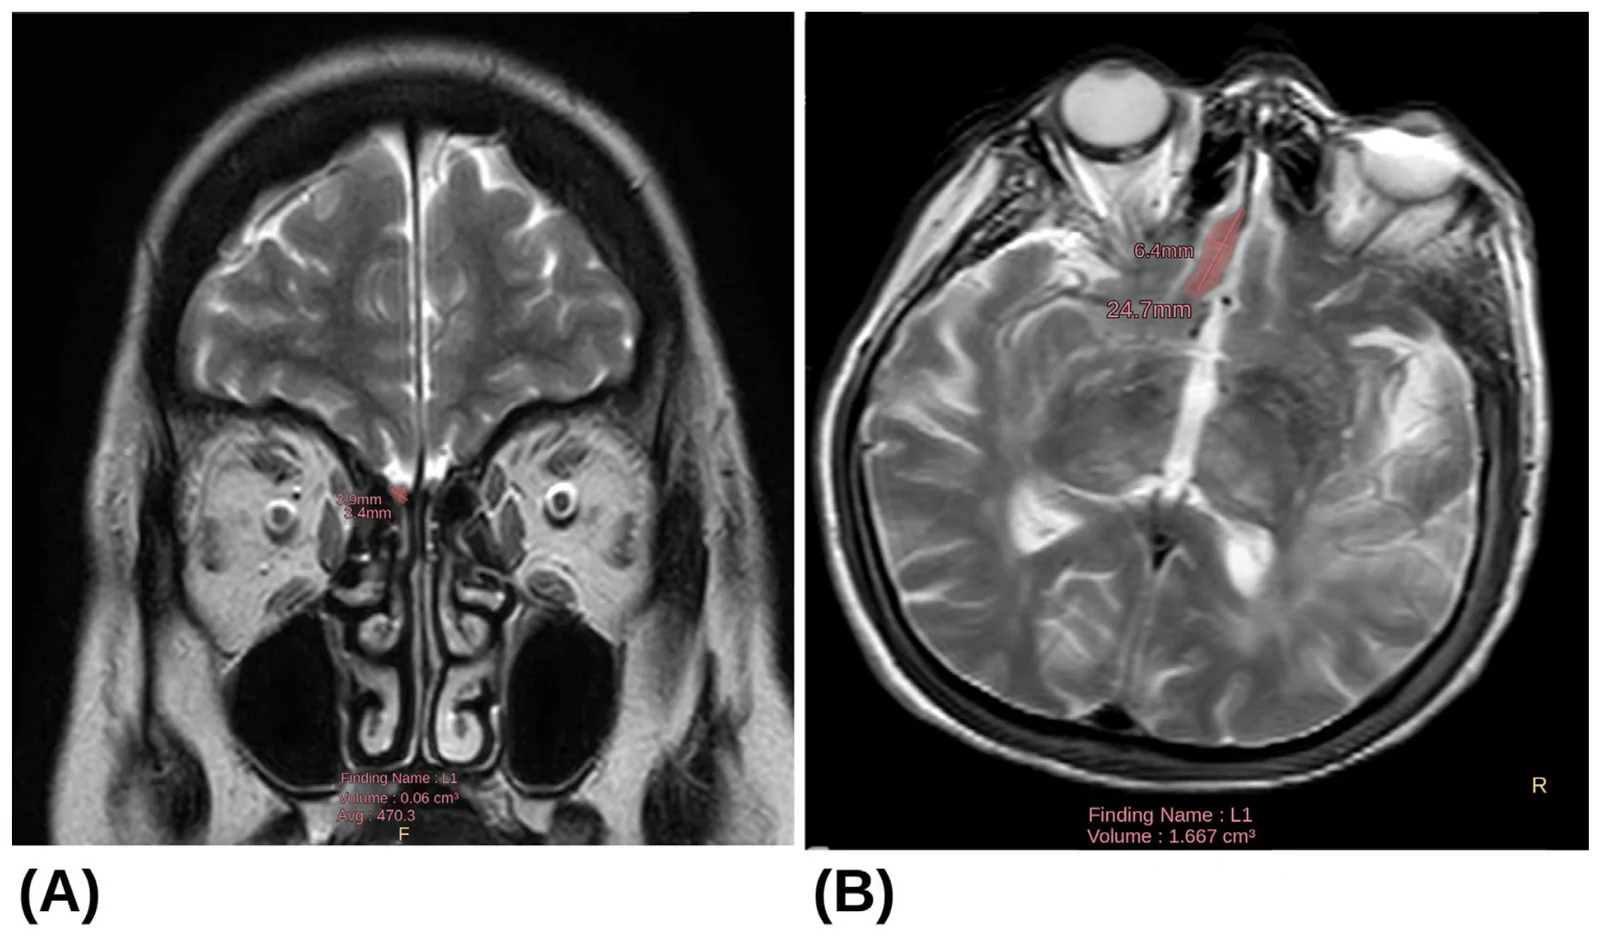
MRI-based volumetric assessment of the olfactory bulb and gyrus rectus. (A) Measurement of olfactory bulb volume on a T2-weighted coronal MRI image. (B) Measurement of gyrus rectus volume on a T2-weighted axial MRI image.
